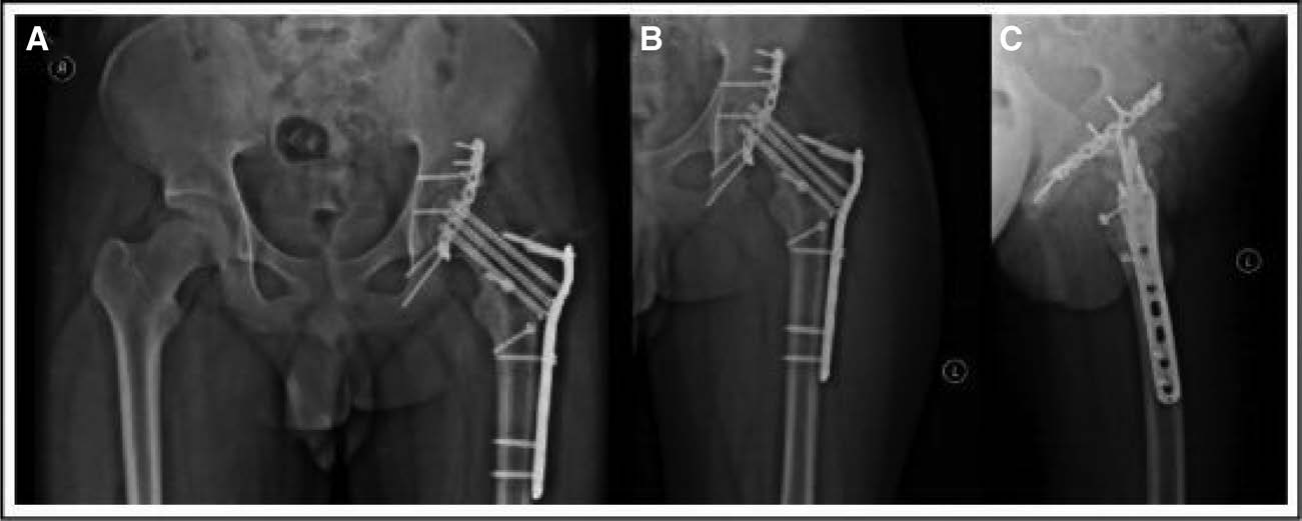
Three months after the operation. (A) The patient review showed that the internal fixation position was consistent with that after the operation, with no collapse and necrosis of the femoral head, no sclerosis of the acetabulum, and a small amount of callus formation, and the fracture line became more blurred than before. (B) Anteroposterior DR image of the left hip joint; (C) lateral DR image of the left hip joint. DR = digital radiography.
